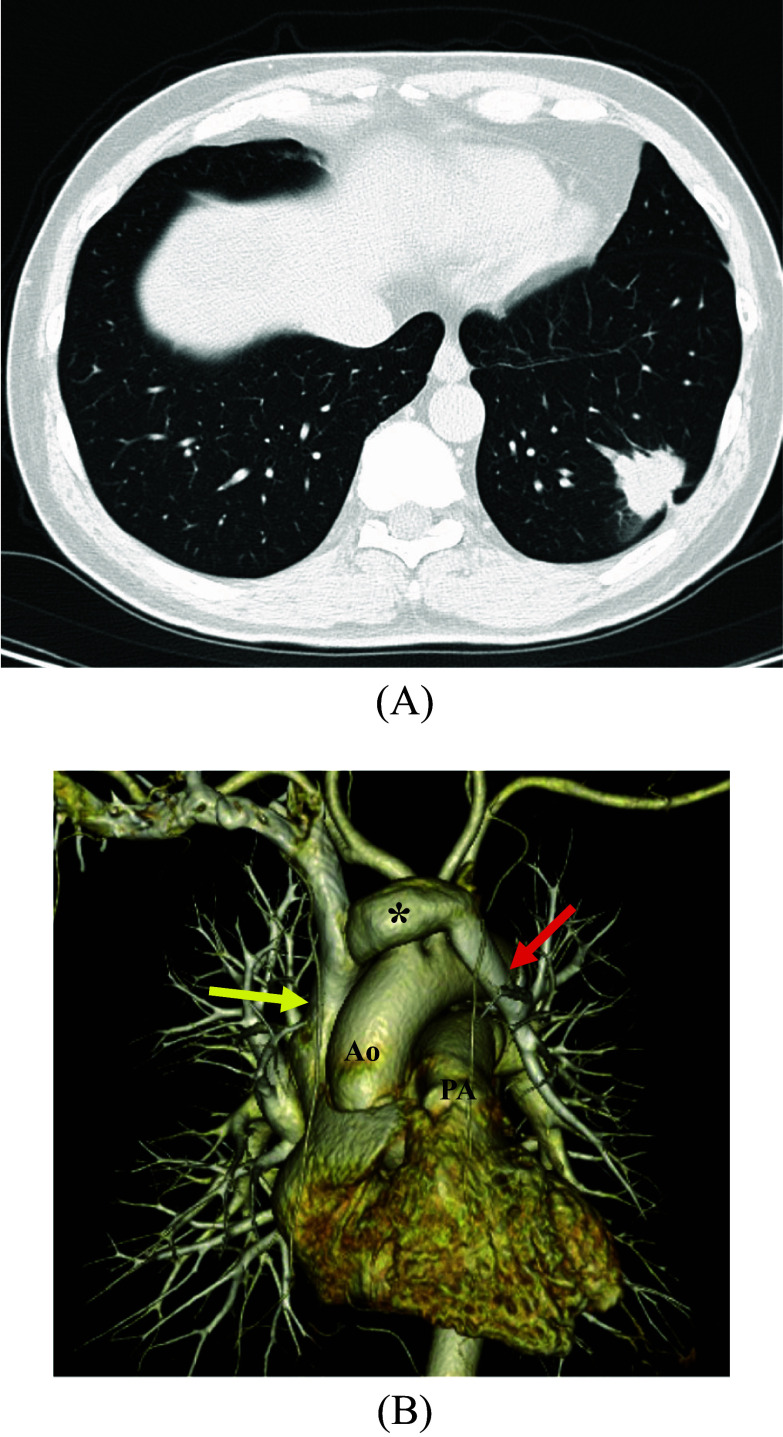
Preoperative contrast-enhanced computed tomography. A Mass with a specula was observed in the left lower lobe. Its border was irregular, and its diameter was ~ 39 mm. B Left upper pulmonary vein (red arrow: LUPV) drained into the superior vena cava (yellow arrow) via the left brachiocephalic vein (asterisk) (*Ao* aorta, *PA* pulmonary artery)
